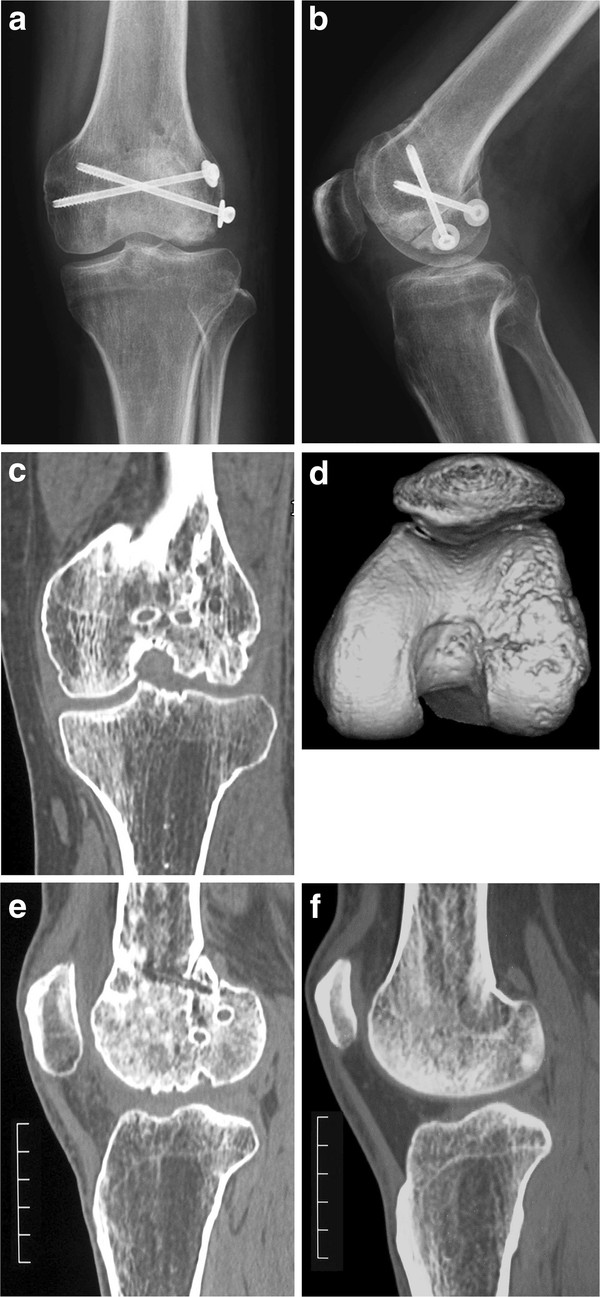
Anteroposterior (a) and lateral (b) radiographs after corrective osteotomy. Computed tomography scans (c-e) at 1.5 years after corrective osteotomy shows complete bony union. Computed tomography scan (f) of the contralateral side and f scale bar: 5 cm.
